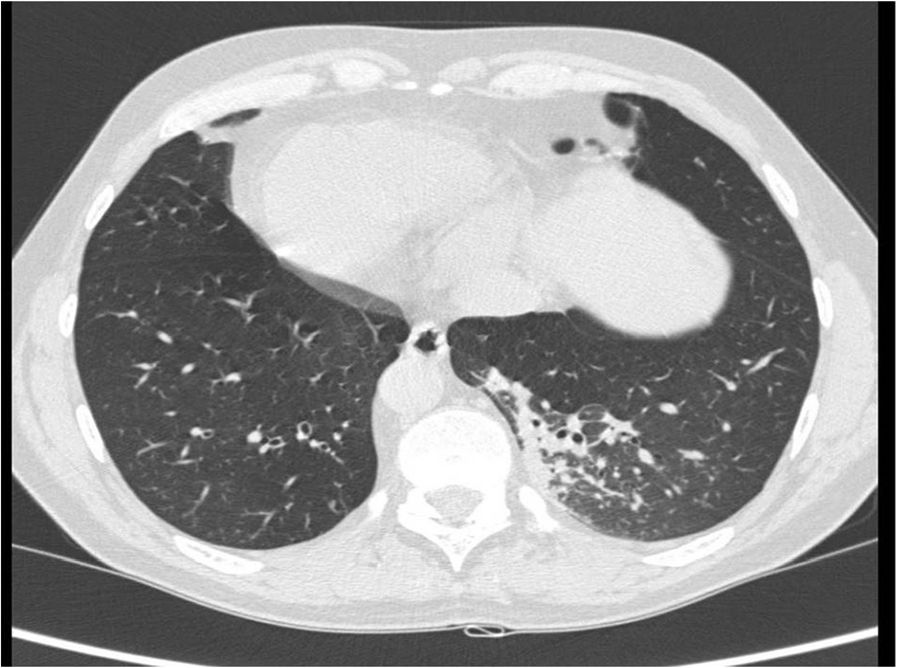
CT-scan performed in patient #3 during hospitalization for pneumonia in lower left lobe.
